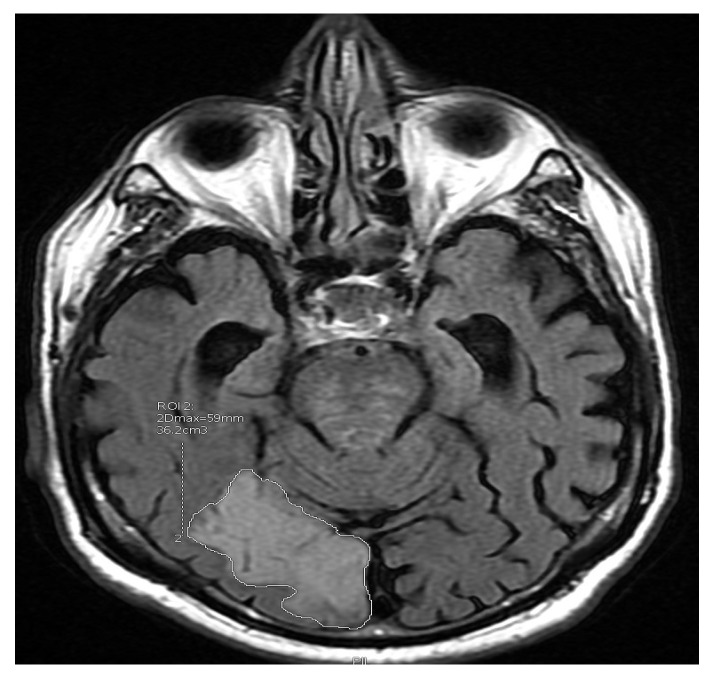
Volumetric evaluation of ischemic focus size in magnetic resonance (MR) in fluid-attenuated inversion recovery (FLAIR) sequence.
